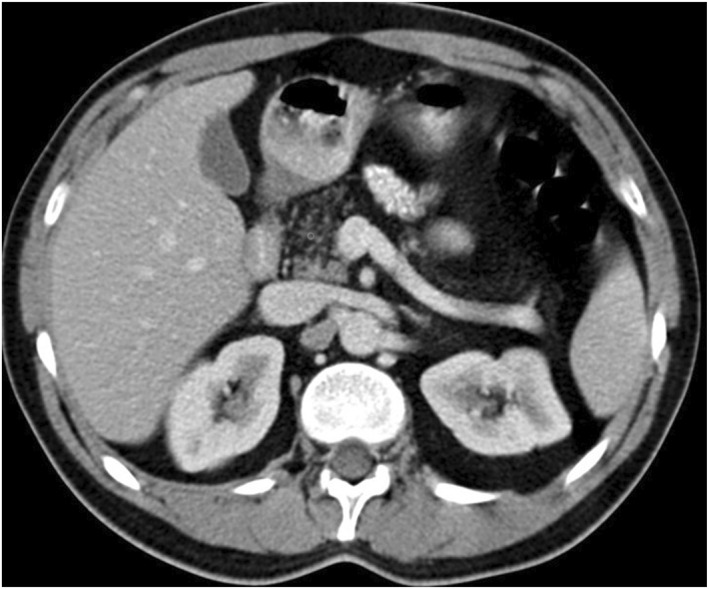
Diffuse fatty pancreas on computed tomography is more evident in the tail and the body than in the head.
